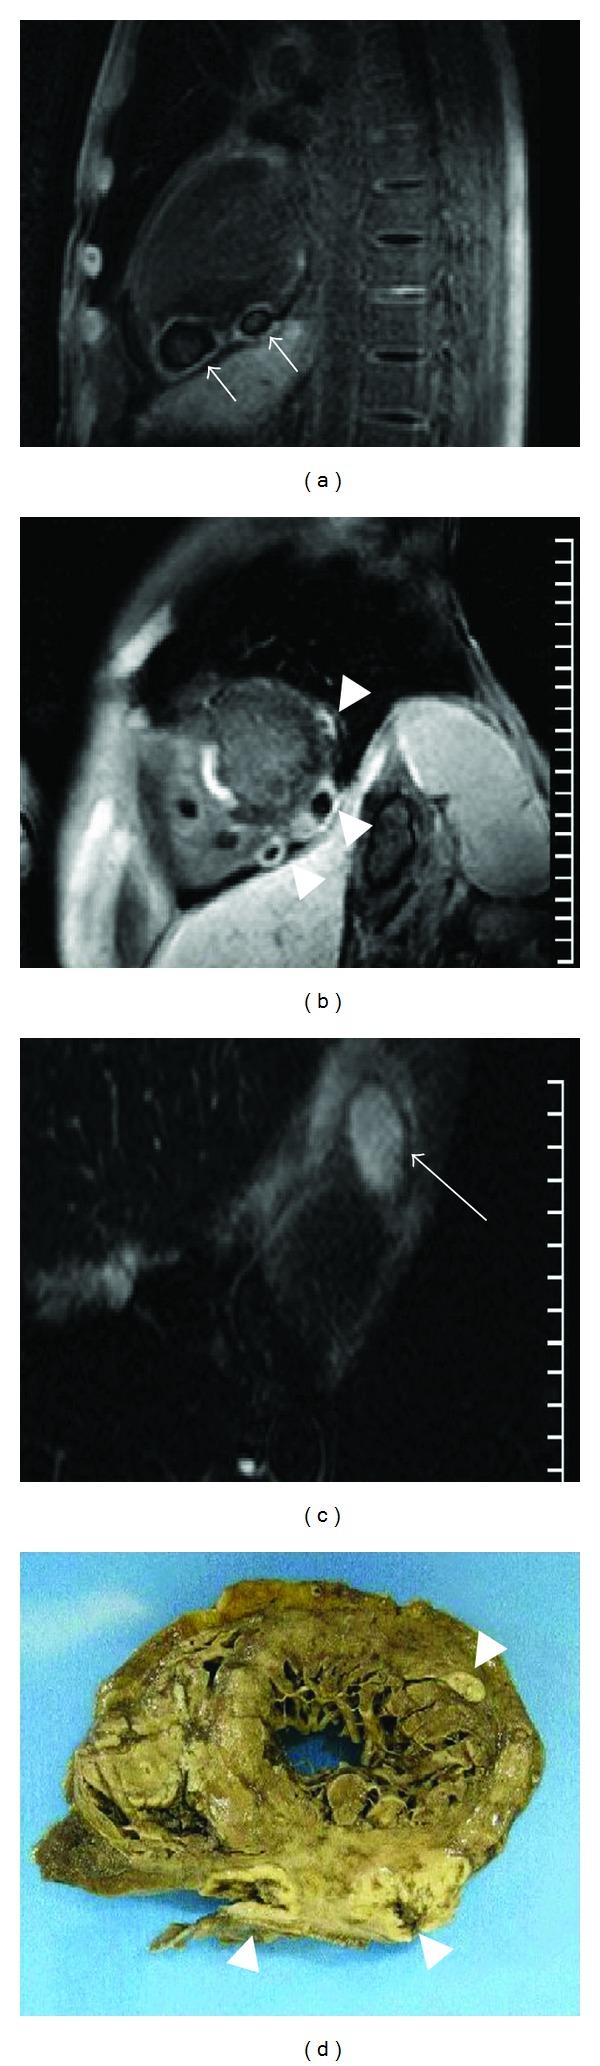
Cardiac MRI performed 6 months after diagnosis and autopsy study. (a) T1-weighted postgadolinium MRI—long axis view. Two collections (white arrows) with thick enhancing rim and hypointense core in the epicardium and myocardium of the left ventricle posterior wall. (b) T1-weighted postgadolinium MRI—short axis view. Lesions (arrow heads) in the left ventricle wall. A hyperintense calcified lesion located in the interventricular septum is also visualized. (c) T2-black blood MRI—axial view. Hyperintense core suggesting a cystic core (black arrow). (d) Autopsy study. Heart short axis view pointing out the three left ventricle abscesses observed on image (b) (arrow heads).
